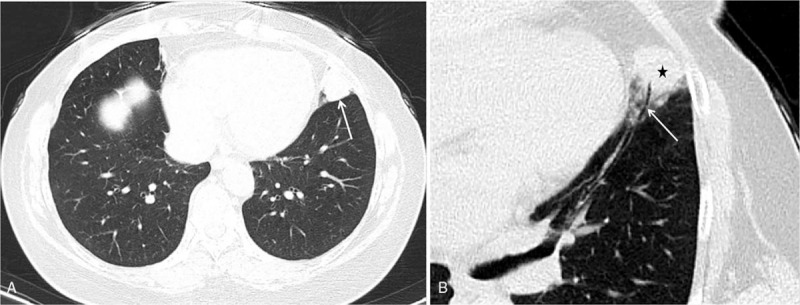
Unenhanced thoracic CT images in a 65-year-old woman with PLELC. The lung window shows a 2.1 × 1.8 cm peripheral nodule (A, arrow) in contact with the visceral pleura, exhibiting a positive lobulation sign (B, black star) and air bronchogram (B, arrow). CT = computed tomography, PLELC = pulmonary lymphoepithelioma-like carcinoma.
